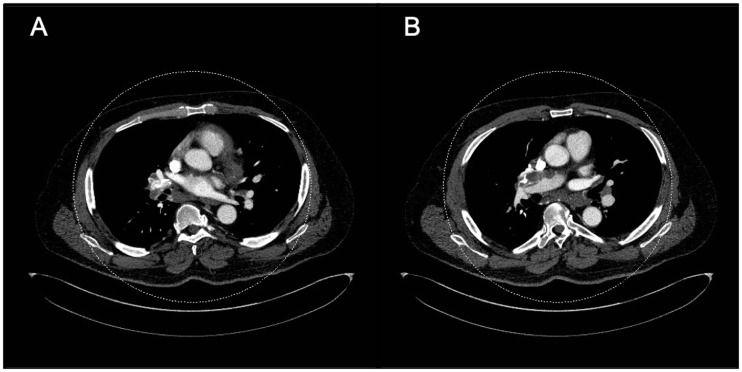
Axial CT images in the mediastinal window settings demonstrate calcified
